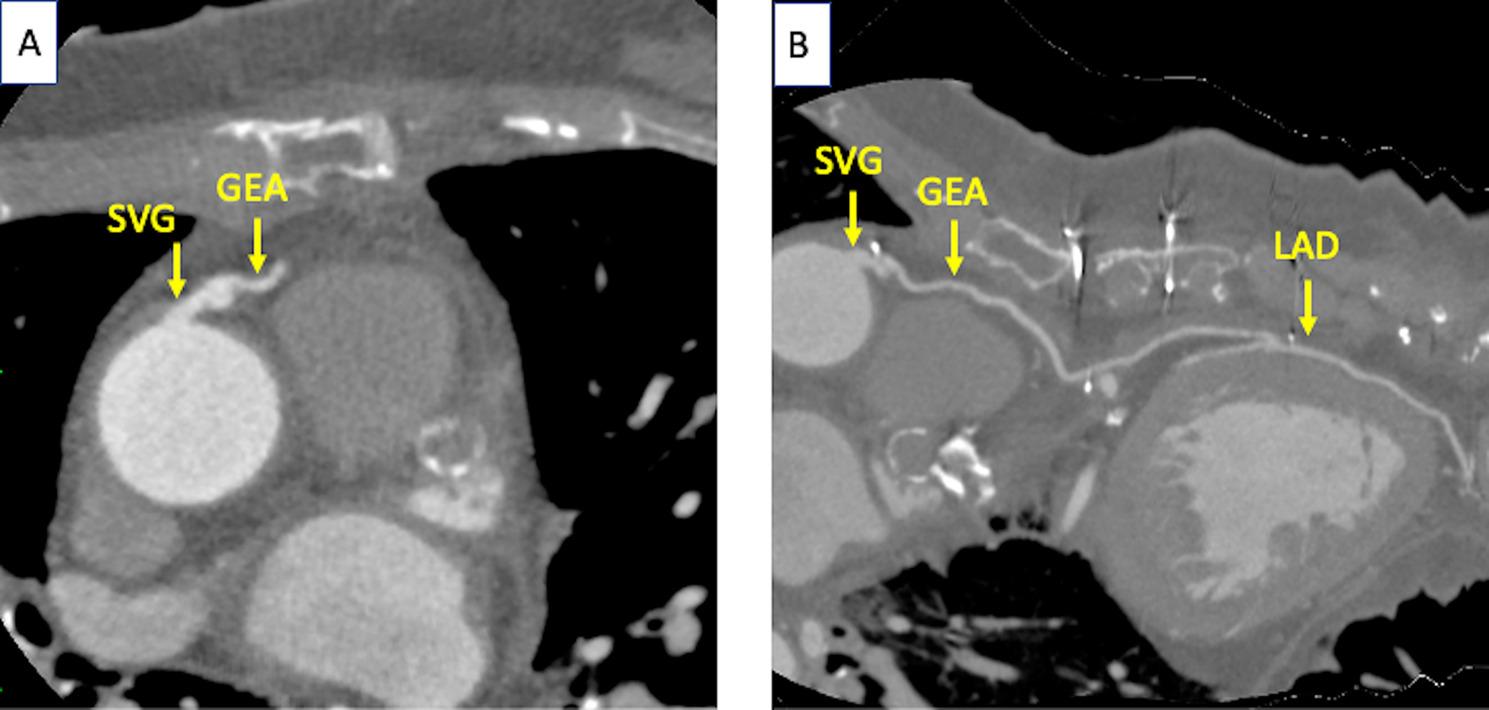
Postoperative contrast-enhanced computed tomography. (A) Axial image at the level of the proximal piggyback anastomosis showing patent SVG and GEA grafts without stenosis. (B) Curved planar reconstruction along the aorta–SVG–GEA–LAD axis, confirming graft patency throughout its course.GEA, gastroepiploic artery; LAD, left anterior descending artery; SVG, saphenous vein graft
